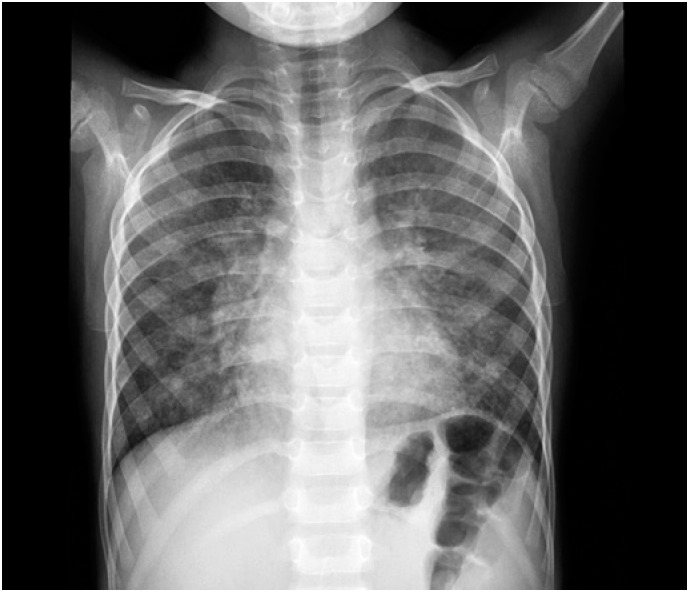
Chest X-ray. Diffuse bilateral opacity with ill-defined edges, with a predominantly central location
